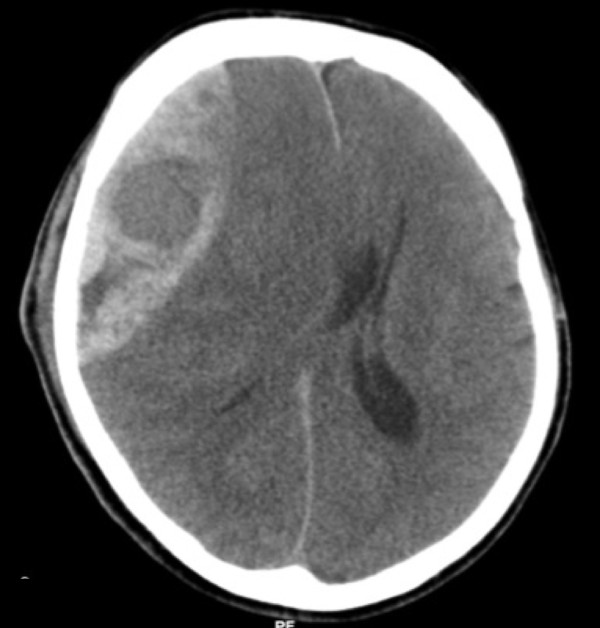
CT scan demonstrating an extradural haematoma.
